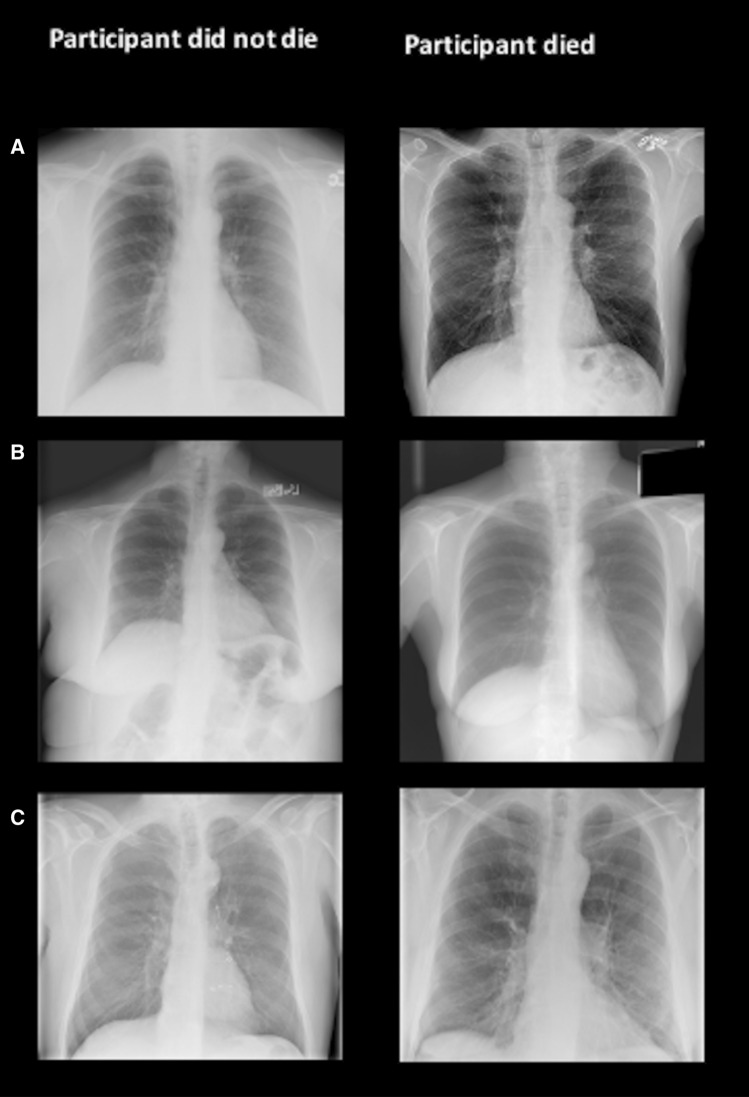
Representative cases from the training data set: in the training data set, image findings (diagnostic and subclinical) as well as gestalt of the image (defined as the degree of deviation from an unremarkable chest radiograph associated with the likelihood of 6-year mortality rated on a binary scale) were assessed. In row (A), both participants presented with emphysema, indicating that a single major diagnostic finding are not reliable predictors for outcome. In row (B), 13 findings were reported in image on left, 4 findings were reported in image on right; example that sum of findings per subject was not associated with mortality. In row (C), image on left surgical clips indicate elevated probability of dying, however, radiologists rated gestalt of the image as “absent” on left and as “present” on right.
